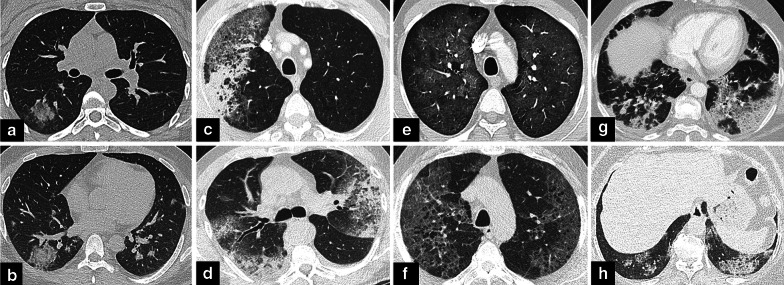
Other infectious diseases that COVID-19 pneumonia may mimic. a Axial CT image shows a consolidation with rounded morphology in the right lower lobe. Hemophilus influenza was detected in the respiratory panel. b Similar round consolidation is observed in the right upper lobe. The patient was tested positive on RT-PCR for COVID-19 pneumonia. The respiratory panel was negative for other causes. c Axial CT image shows GGOs with associated consolidations in a patient with Hemophilus influenza pneumonia. d In a patient diagnosed with COVID-19 pneumonia, bilateral GGOs with consolidations are observed on axial CT image similar to (c). e Axial CT image shows GGOs in bilateral upper lobes in an immunosuppressed patient compatible with PJP infection. f Bilateral upper lobe GGOs in a patient diagnosed with COVID-19 pneumonia. GGOs superimposed on emphysematous parenchyma may interfere with PJP. Subpleural sparing is in favor of PJP. g Axial CT image shows bilateral consolidative opacities in lower lobes in a patient diagnosed with influenza A pneumonia. h In a patient diagnosed with COVID-19 pneumonia, bilateral opacities are observed in lower lobes
